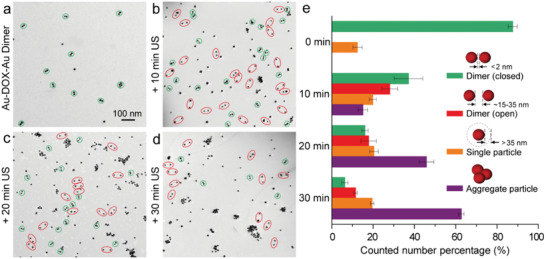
US‐induced structural change of Au‐DOX‐Au dimer nanoswitches. Representative TEM images of a) Au‐DOX‐Au without ultrasonication, Au‐DOX‐Au with ultrasonication for b) 10 min, c) 20 min, and d) 30 min, respectively (green circled: closed; red circled: open). e) The corresponding number percentage histograms of Au‐DOX‐Au with different morphologies after US treatment for 0, 10, 20, and 30 min, respectively. The statistical analysis was repeated with three independent samples. Mean values: SD from the mean, *N* = 3 independent experiments.
